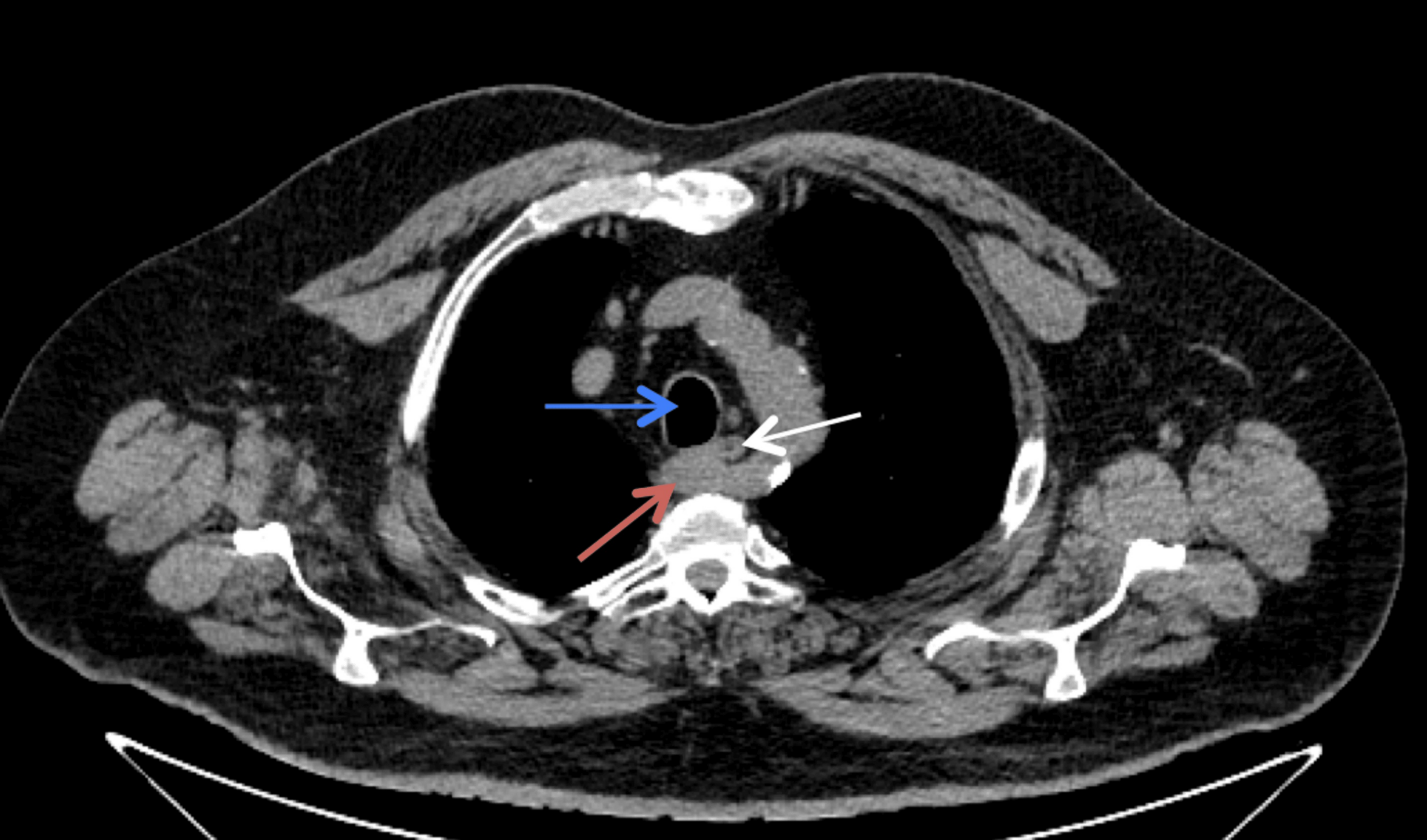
Thoracic CT scan (axial view) showing the aberrant subclavian artery (red arrow), thoracic esophagus (white arrow), and trachea (blue arrow) CT: computed tomography
